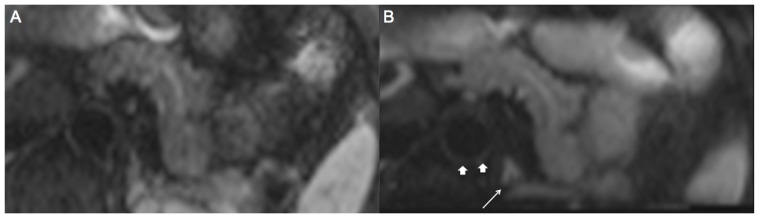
A 47-year old patient with c-EPI DWI (A) and z-EPI DWI (B) of the pancreas. z-EPI DWI demonstrates less distortion and a more homogeneous delineation of the pancreatic body. Also structures adjacent to the pancreas like the adrenal gland (thin arrow) and the aorta (arrows) are better delineated more sharply with z-EPI.
